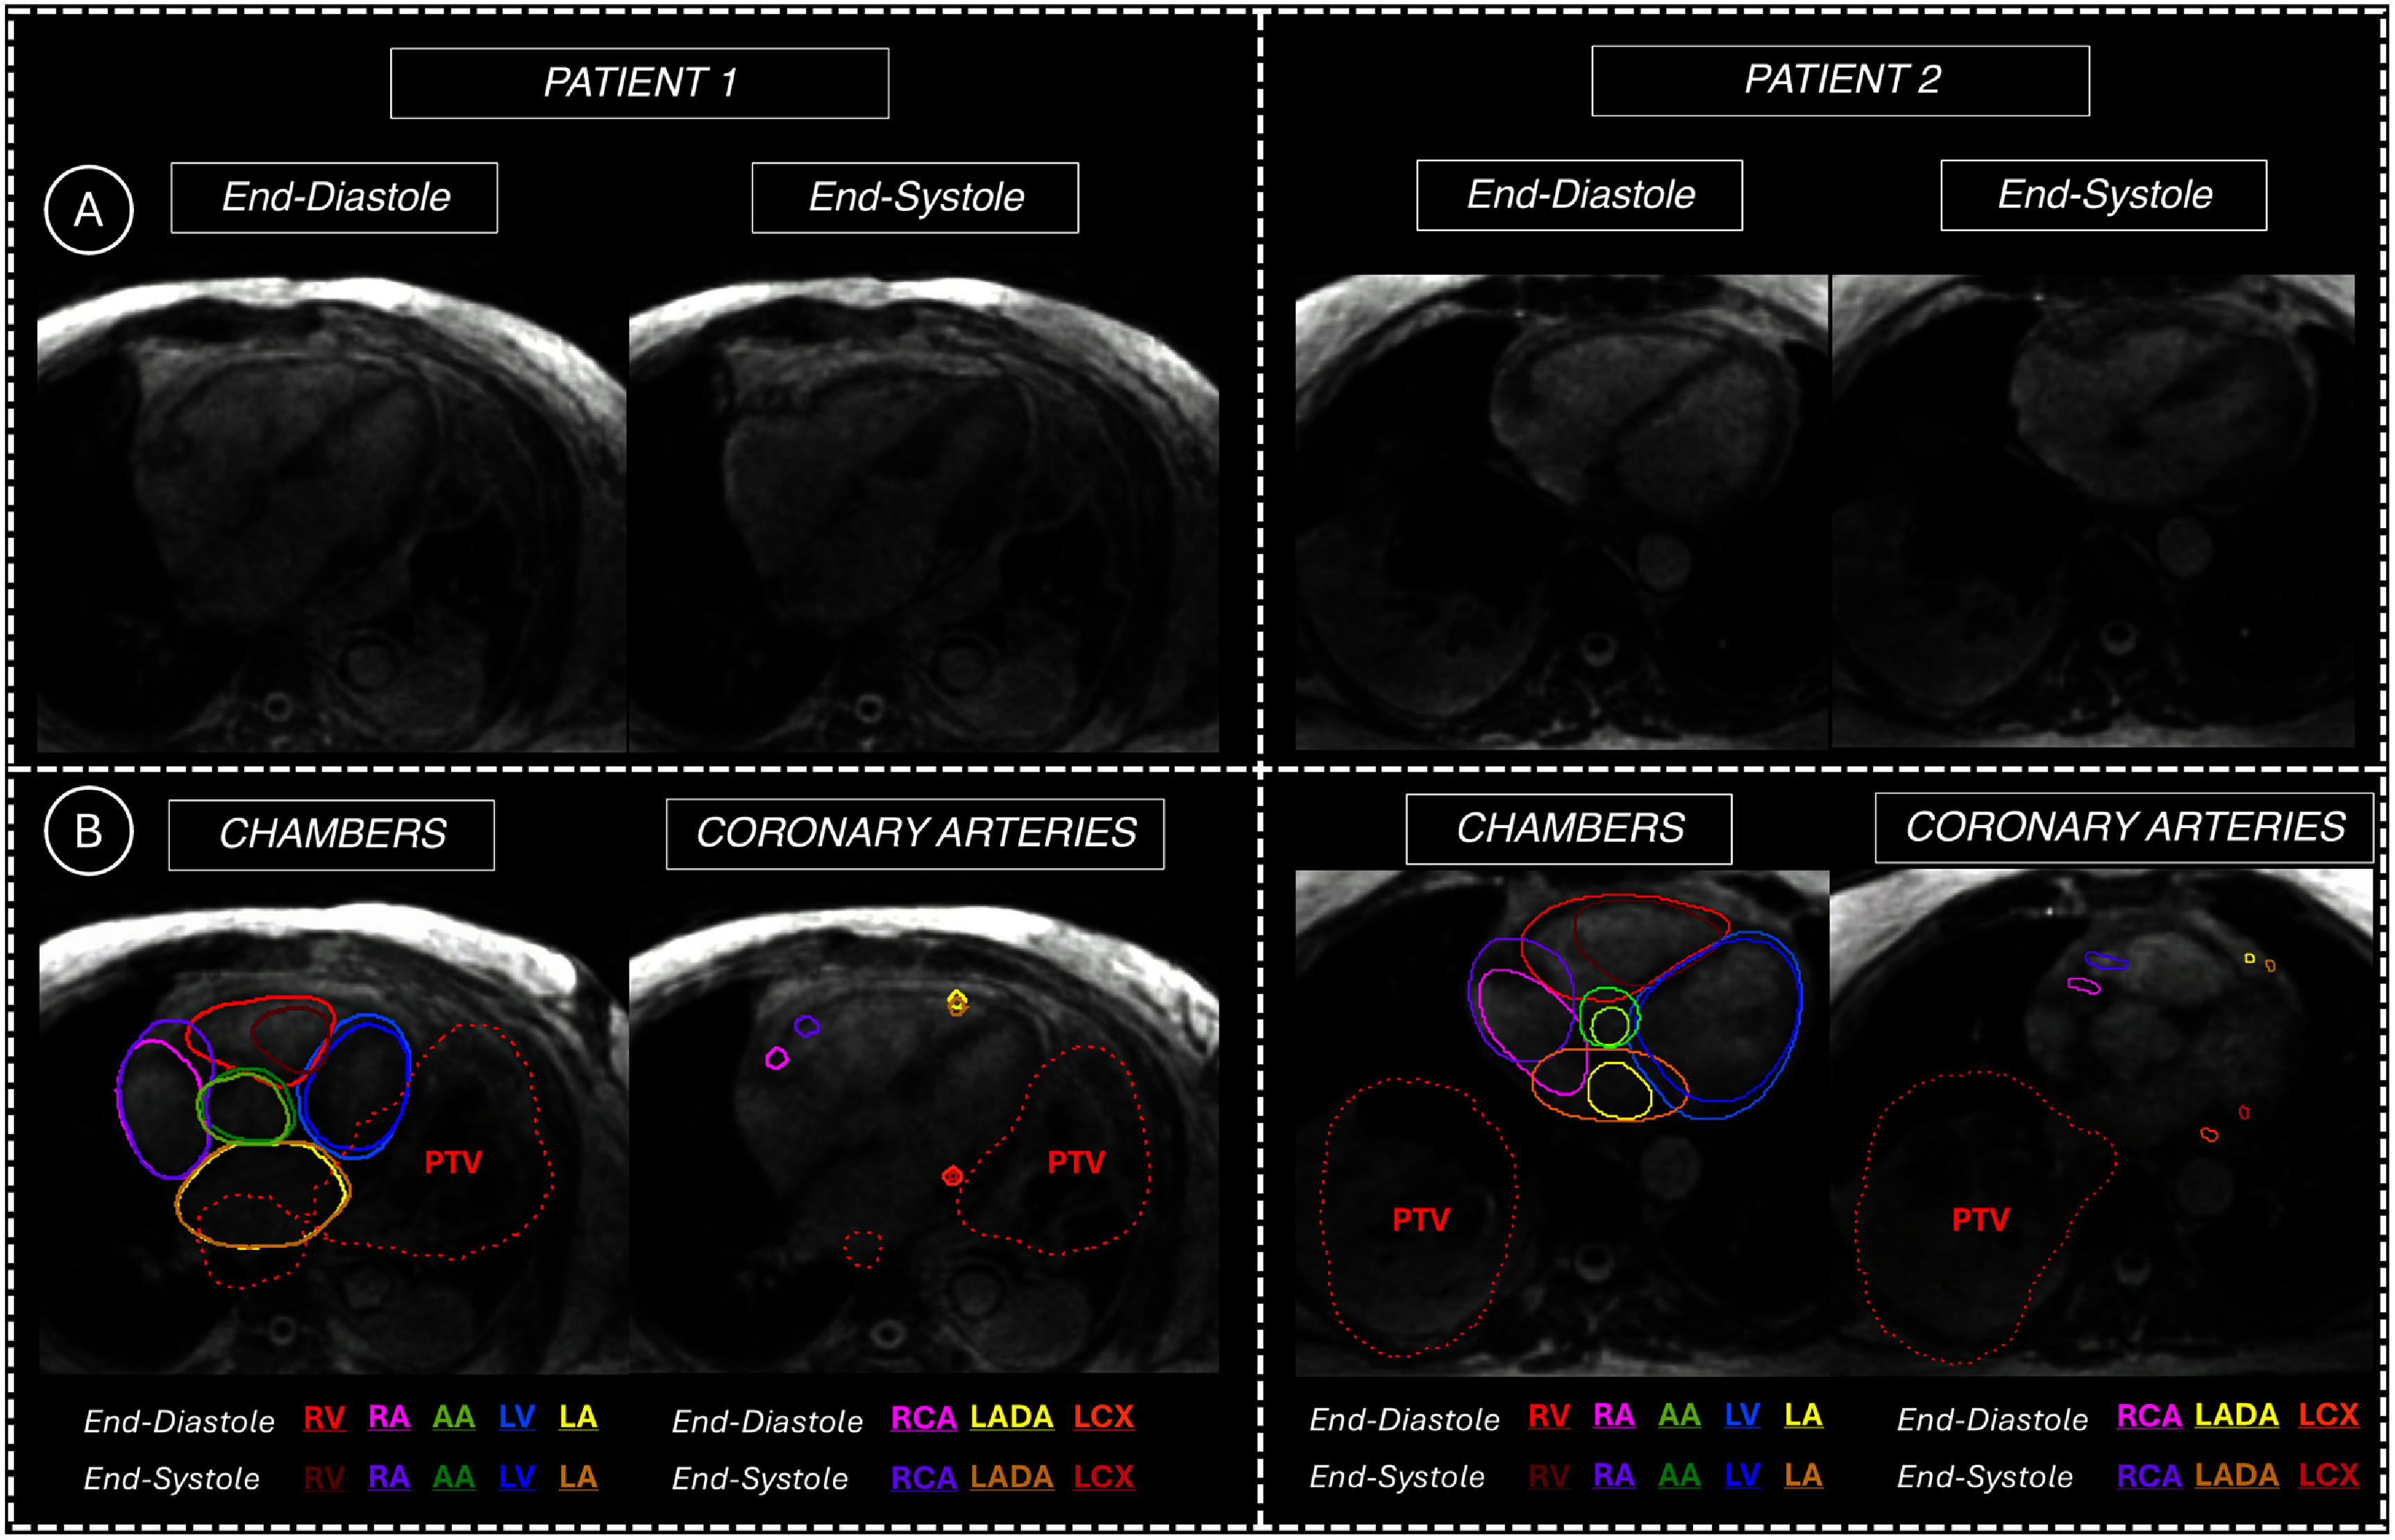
(A) 5D-MRI images acquired from patients with left-sided (left) and right-sided (right) lung cancer at end-inhale for end-diastole and end-systole. (B) Contours for the chambers/ascending aorta and coronary arteries, with the PTV shown with a dashed red line, for both patients.
